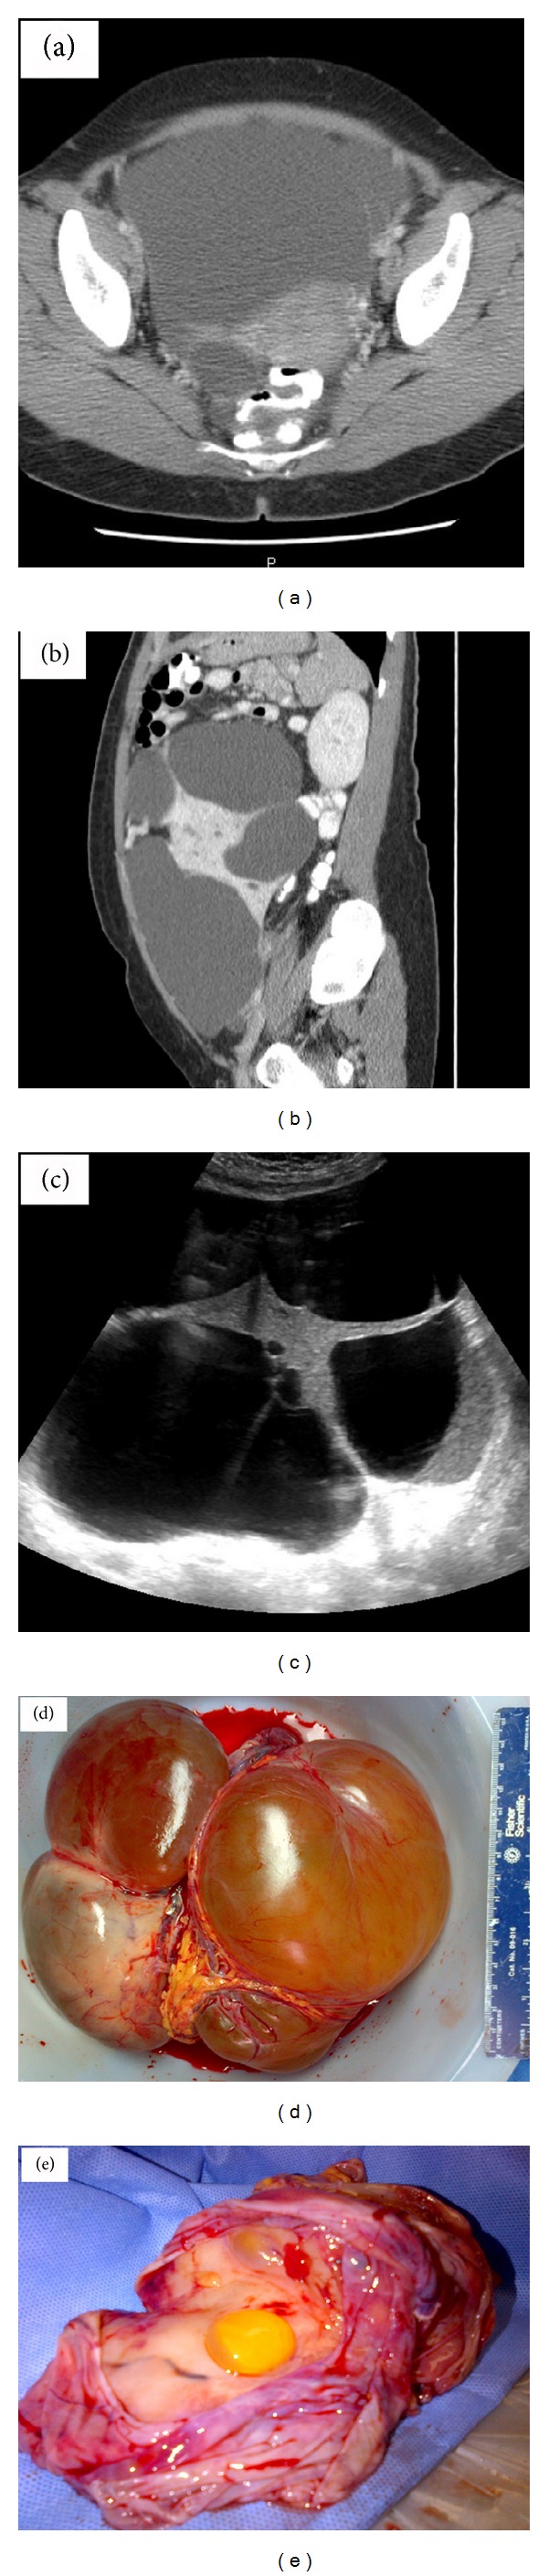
(a) CT image: axial view. (b) CT image: sagittal view. (c) Ultrasound image: transvaginal. (d), (e) Photo: gross pathology.
